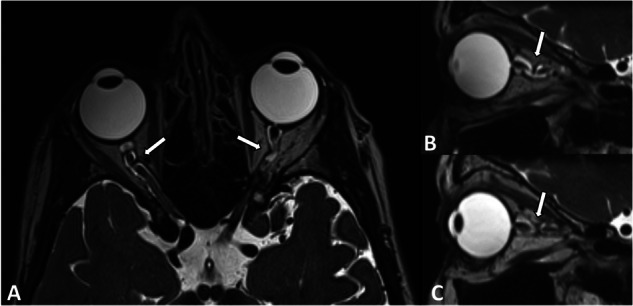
MRI images of a 67-year-old female normal tension glaucoma patient with optic nerve kinks in both planes in both optic nerves. A Kinking in the axial plane. B Kinking in the sagittal plane of the right optic nerve and C Kinking in the sagittal plane of the left optic nerve. Kinking marked with arrows.
